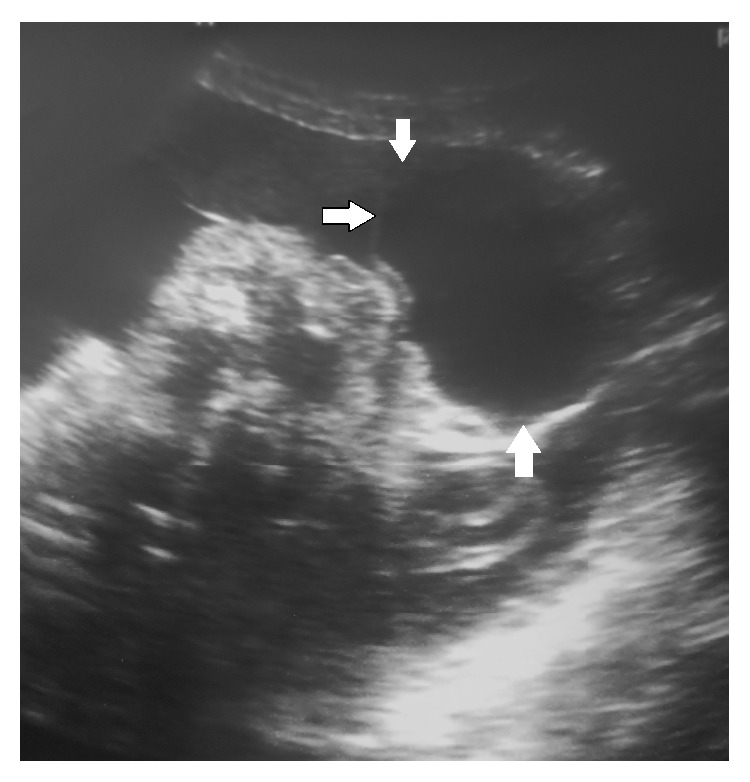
Sonographic examination showing a regularly shaped, midline, facial cystic mass.
